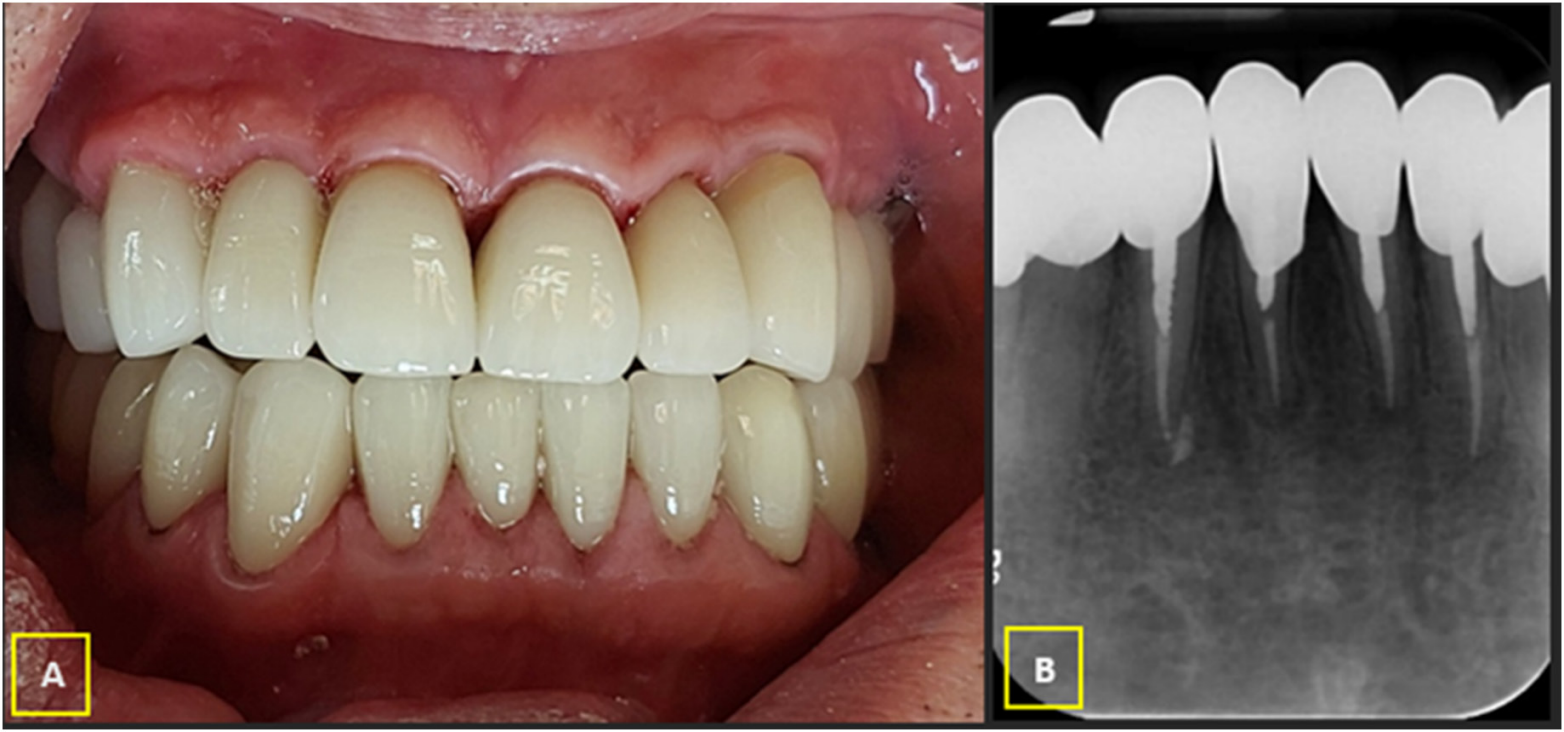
(A) Intraoral photography. (B) Periapical radiograph; At six‐month follow‐up.
